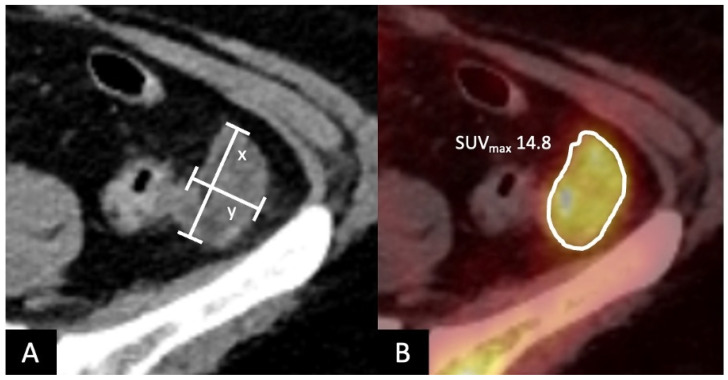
A fifty-two-year-old patient with diffuse large B-cell lymphoma (DLBCL) and abdominal lymphoma manifestation left-sided in the paracolic channel, lateral to the descending colon. Example of image analysis with (A) measurement of maximal- (x) and minimal- (y) diameter in mm to determine tumor volume and (B) measurement of the SUV_max_ in the tumor area with the highest metabolic activity using a volume of interest (VOI).
